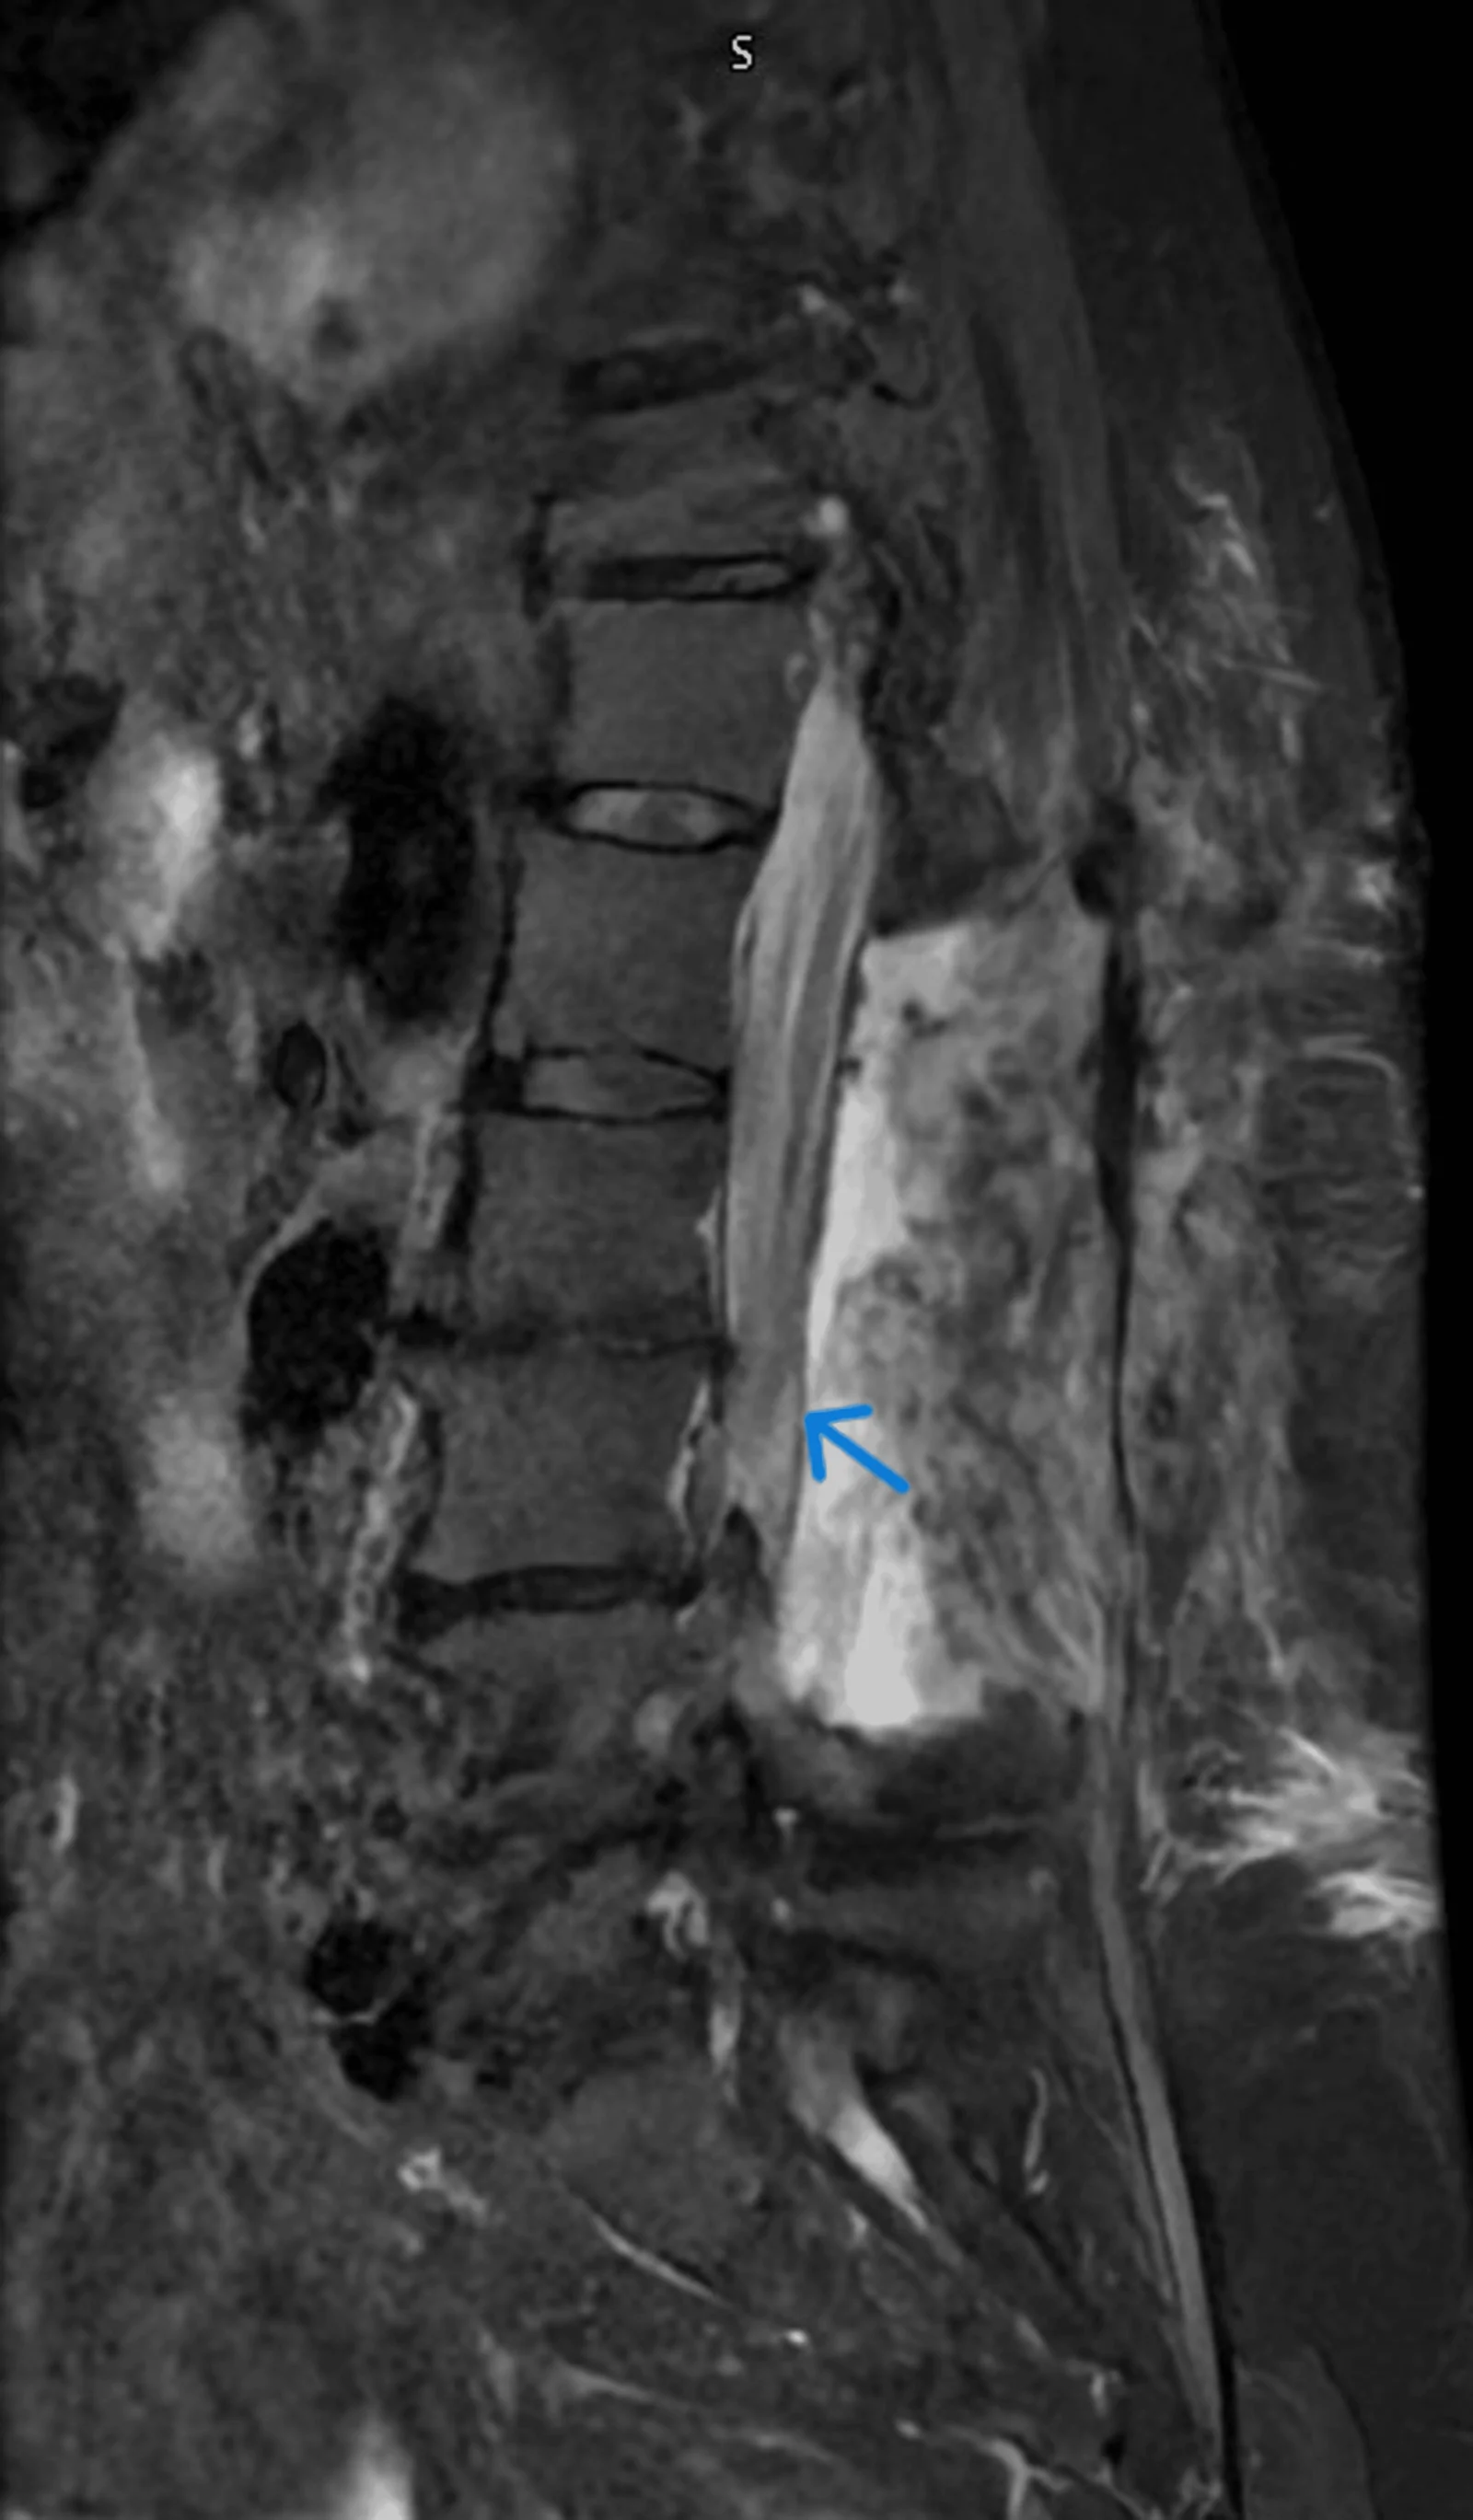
Postoperative sagittal T2‑weighted MRI of the lumbar spine Postoperative sagittal T2‑weighted MRI at two weeks demonstrating complete evacuation of the subdural hematoma and restoration of thecal sac contour (arrow).
